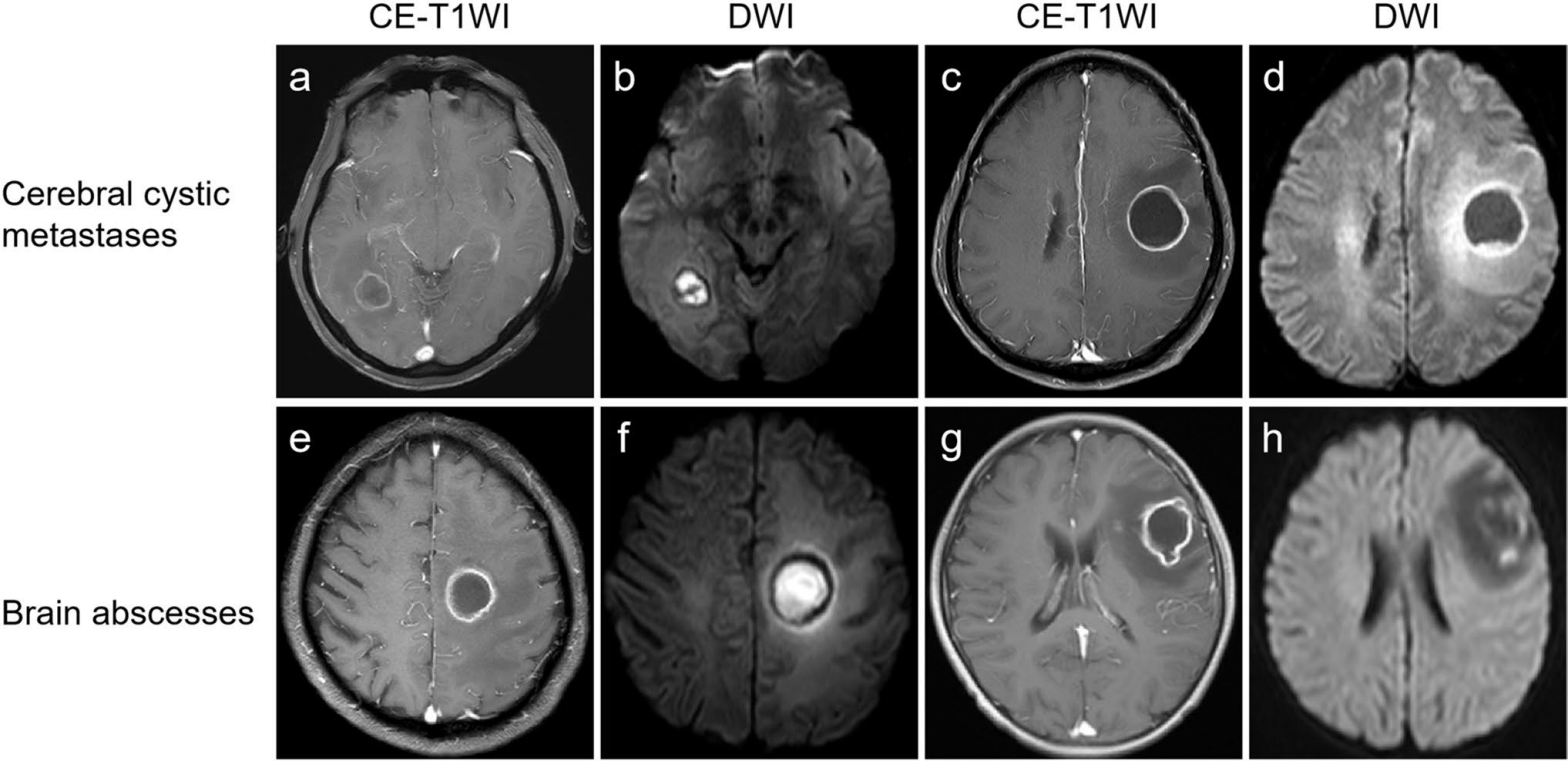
Representative examples of cerebral cystic metastases and brain abscesses of the capsular stage. Cerebral cystic metastases (a–d) and brain abscesses of the capsular stage (e–h) exhibit similarly rim enhancing on CE-T1WI. a, b A 51-year-old male with cerebral cystic metastasis from lung adenocarcinoma. The core of the lesion presented hyperintensity on DWI. c, d A 56-year-old male patient with cerebral cystic metastasis from esophageal carcinoma. The core of the lesion presented hypointensity on DWI. e, f A 58-year-old male with bacterial brain abscess. The core of the lesion presented hyperintensity on DWI. g, h A 9-year-old male patient with fungal brain abscess. The core of the lesion presented hypointensity on DWI
